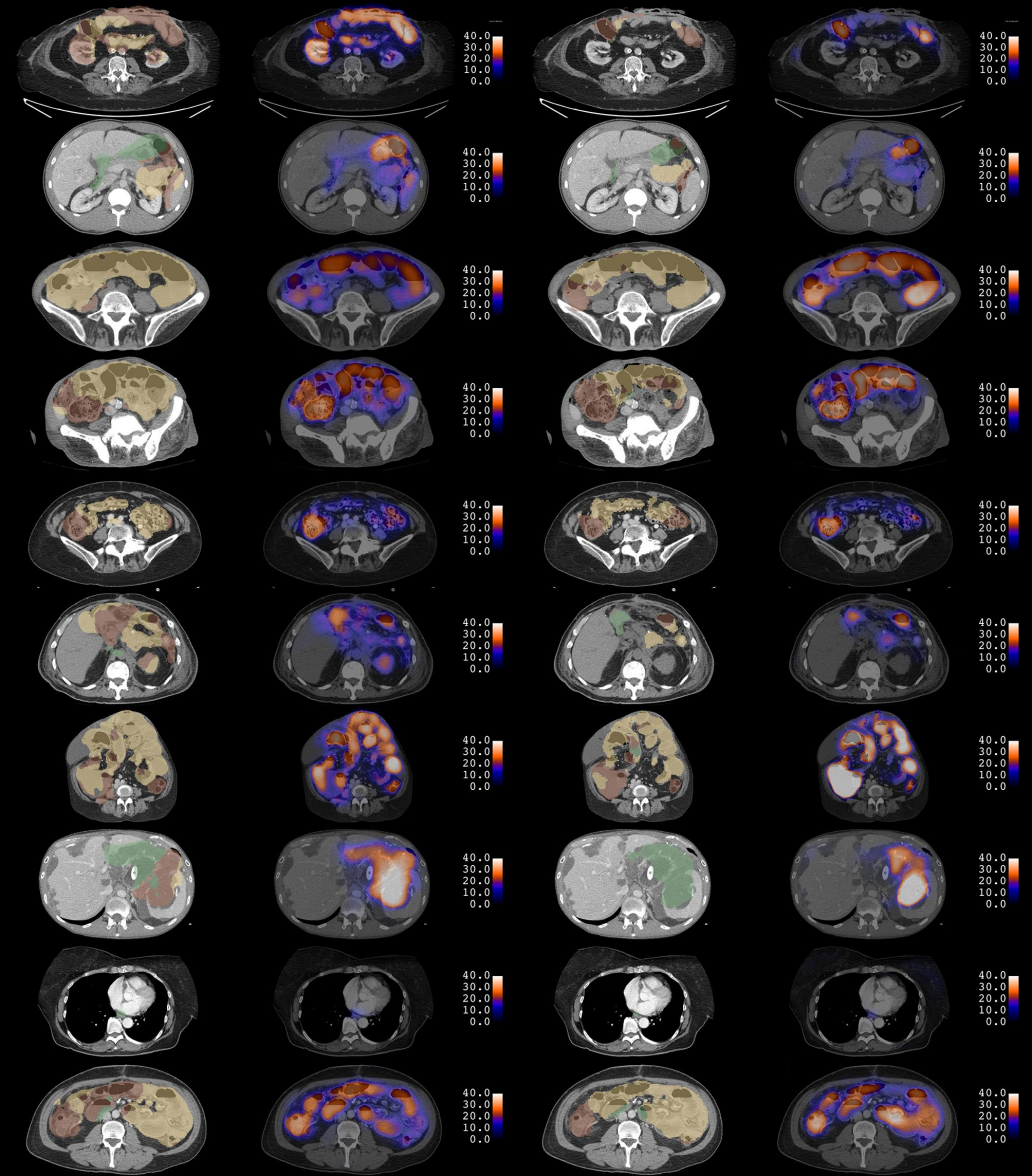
Examples of CNN predictions in 10 test subjects. Each row corresponds to the same single slice of a CT scan as in Fig. 3. 2d CNN predictions for bowel segmentation and diameter maps are shown in the first and second columns. 3d CNN predictions are shown in the third and fourth columns. In both segmentations, red, yellow, and green correspond to foregut, midgut, and hindgut, respectively. Diameters are expressed in units of millimeters. Some incorrect predictions of features are noted
